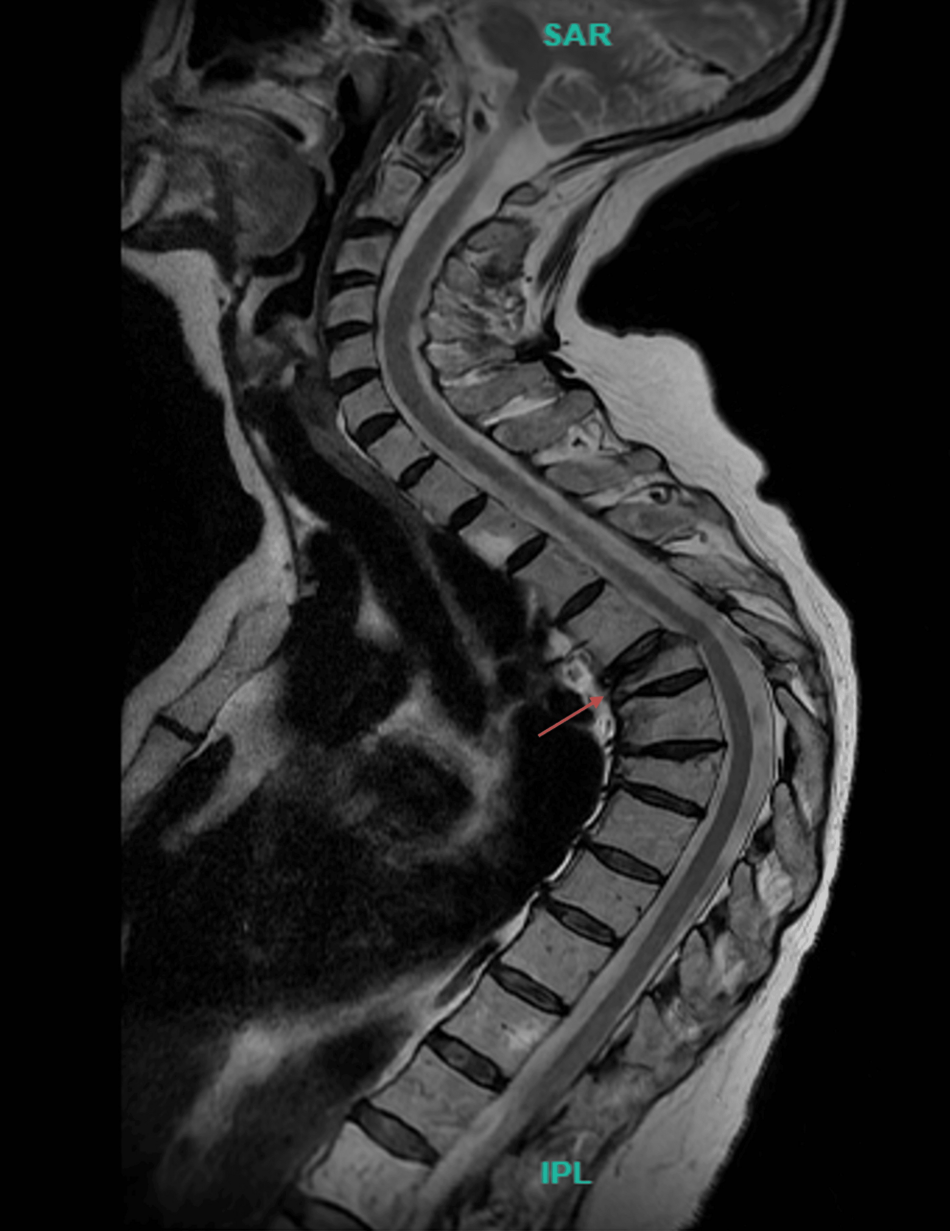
MRI indicating a compression deformity at the T5 vertebral body level, with a 75% reduction in height (red arrow)
